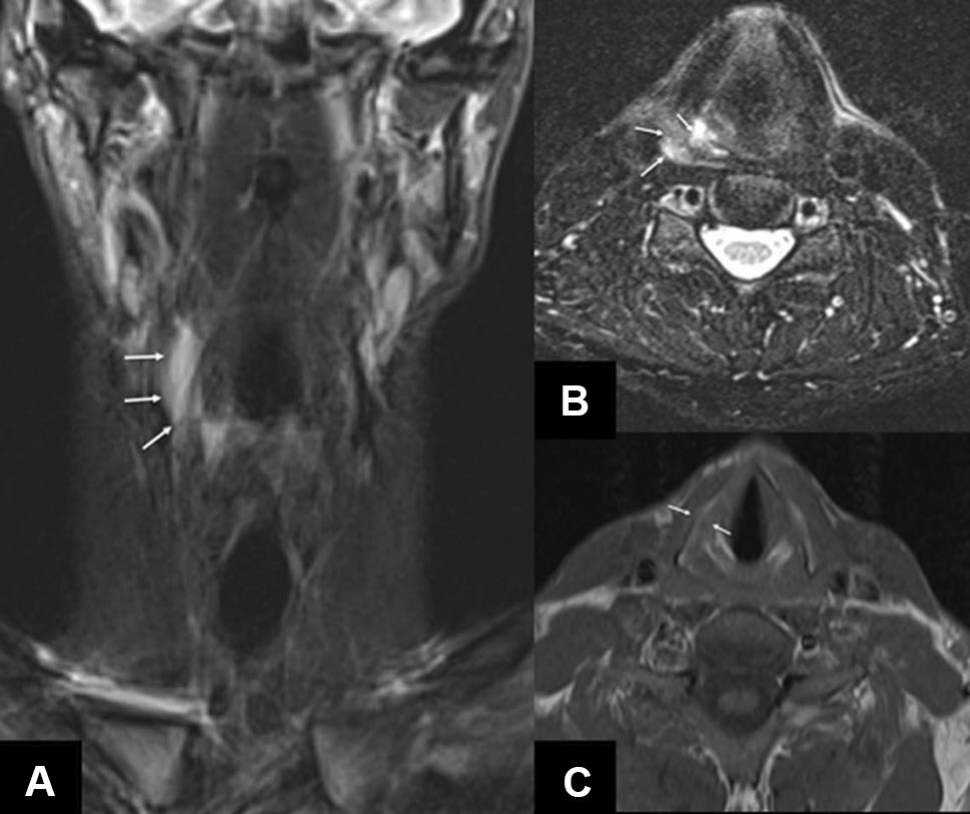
Non-enhanced MRI scan of the neck with a coronal STIR (ST 4 mm), an axial T2-weighted image with fat suppression (ST 3 mm) and a T1-weighted image (ST 3 mm). The MRI scan shows a marked hyperintense edema of the right-sided supraglottic larynx (arrows in A and B). The arrows in the T1-weighted image (C) point to the undislocated fracture of the left thyroid cartilage
